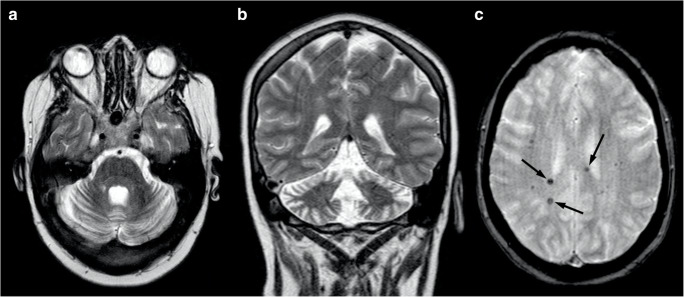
Neuroradiological findings in a 26-year-old female AT patient. A mild global cerebellar atrophy is shown in both axial (a) and coronal (b) T2-weighted images, while the T2*-weighted sequence allows for the depiction of small punctuate hypointense foci (arrows in c) representing both hemosiderin deposits and telangiectatic vessels
